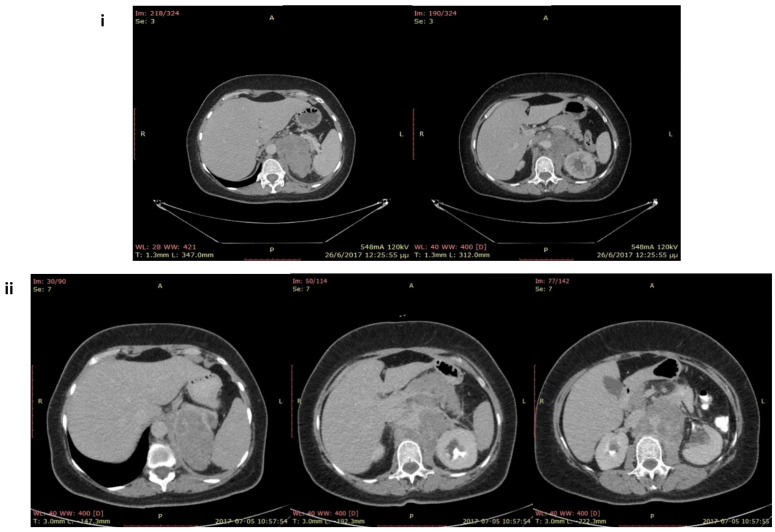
CT scans of the upper and lower abdomen from the first patient: (i) first CT scan (at the initial diagnosis with a max diameter of 8.5 × 7 × 4.2 cm; (ii) second CT scan without contrast in sequential incisions revealed a large left adrenal mass with a max diameter of 8.5 × 10 × 15 cm after 20 days.
